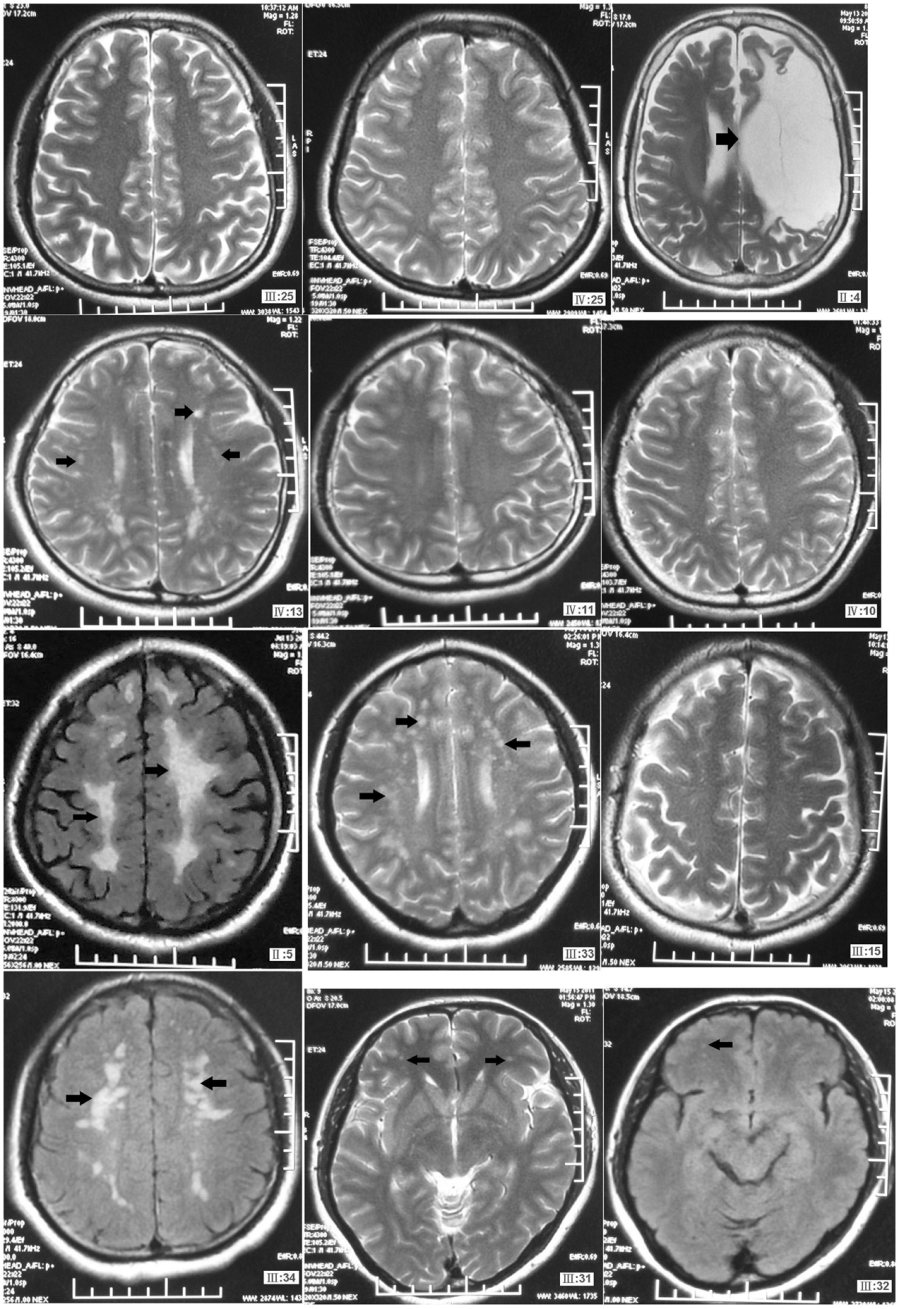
Cerebral MRI examination in the CADASIL family members. ΙΙ:5, the proband, was a 60-year-old male affected family member. ΙΙΙ:31, ΙΙΙ:32, ΙΙΙ:33 and ΙΙΙ:34 were the children of the proband (ΙΙ:5). ΙΙ:5 and ΙΙΙ:32 showed evident clinical features, but ΙΙΙ:31, ΙΙΙ:33 and ΙΙΙ:34 did not show any clinical feature, although they were all mutation carriers (see the long T1 and T2 signals). ΙΙΙ:25, ΙΙΙ:15, ΙV:25, ΙV:10 and ΙV:11 were healthy core family members. ΙΙΙ:4 is a patient with l massive cerebral infarction, who did not have the R133C mutation.
